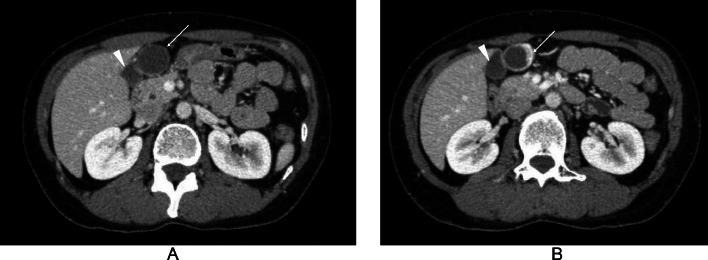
Computed tomography (CT) findings. (A) CT findings could not negate the continuity between the gall bladder (arrowhead) and the cystic lesion (arrow). (B) CT clearly showed an early enhancement pattern in the solid part (arrow) of the intracystic tumor.
